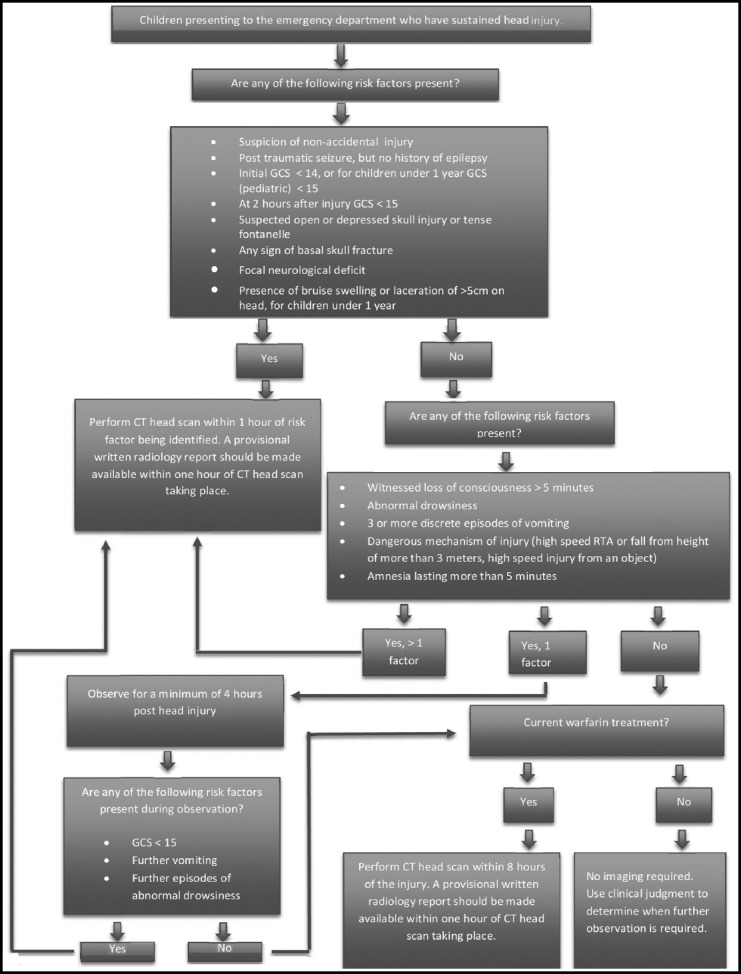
NICE guidelines for selection of children for CT head scan.^11^
